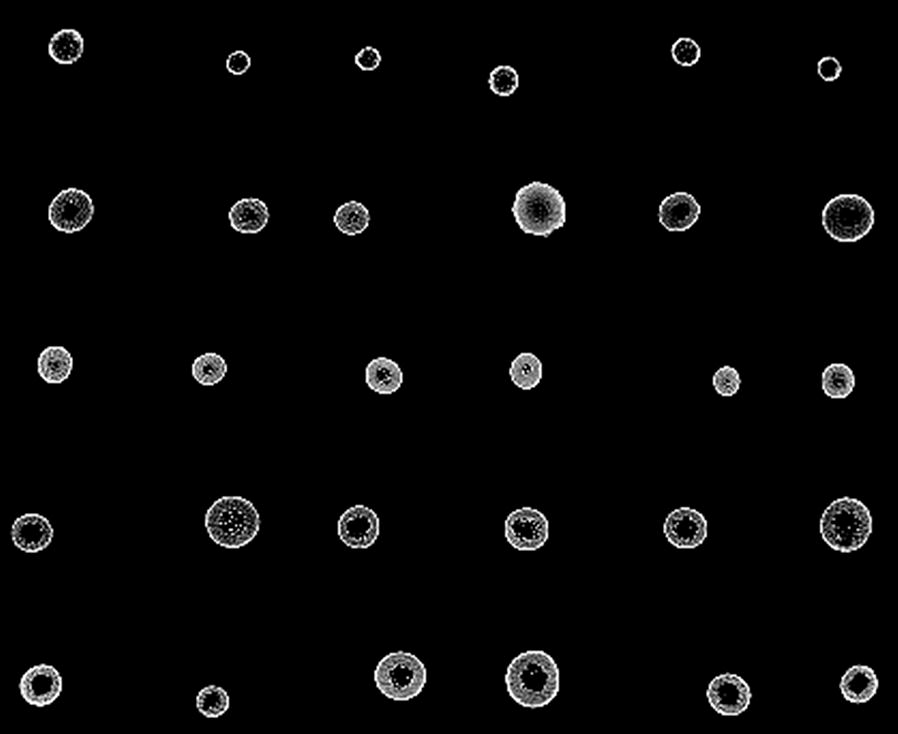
Cross sections of 30 sorghum stems during one run of CT scanning showing different attenuation levels
